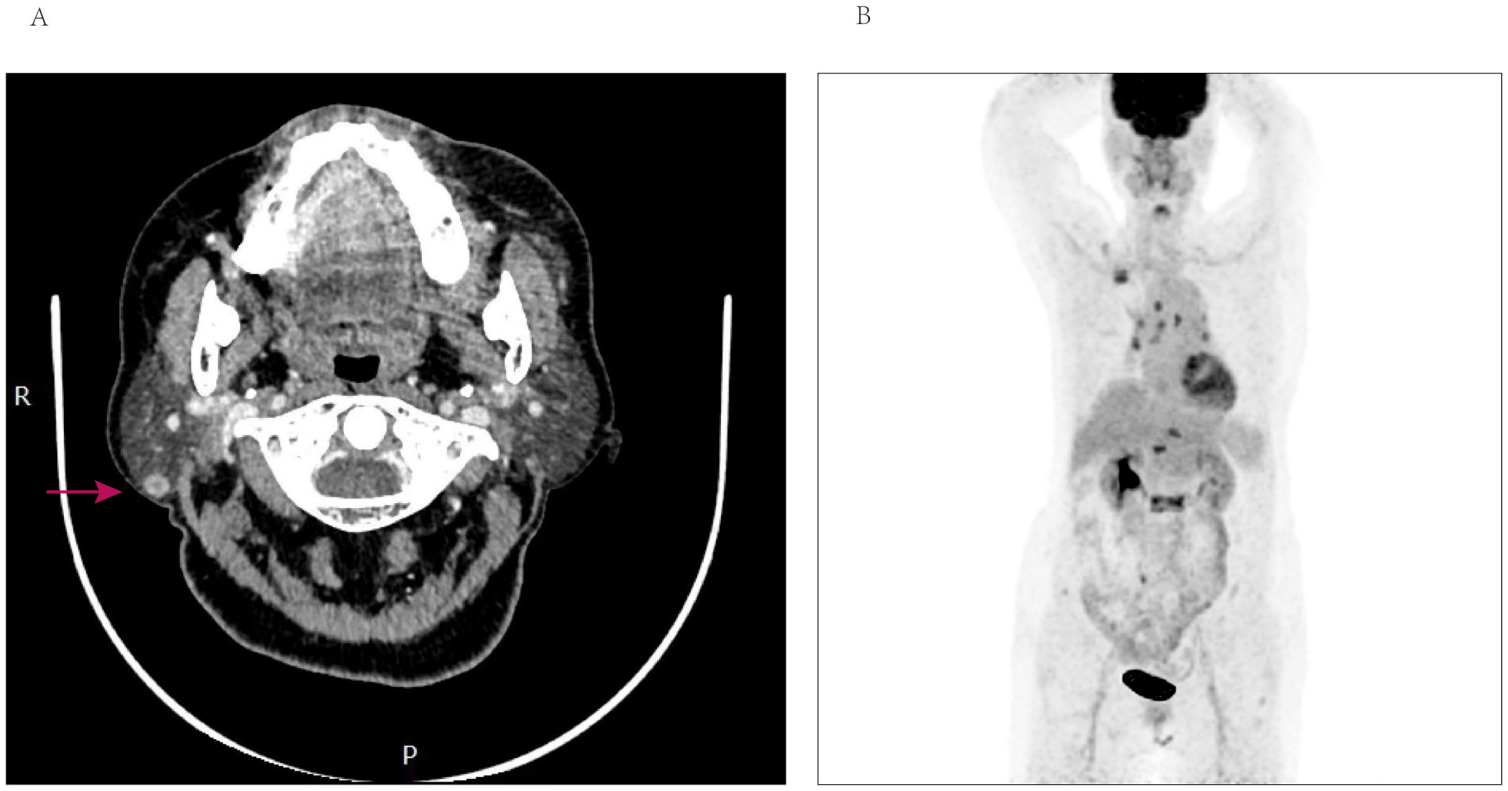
(A) Computed tomography of the parotid with contrast—axial view, demonstrating well-demarcated enhancement nodular of the right parotid gland. (B) PET-CT scan reveals accumulation of SUV more than 8 in multiple vertebrae, ribs, the right ilium, and lymph nodes.
